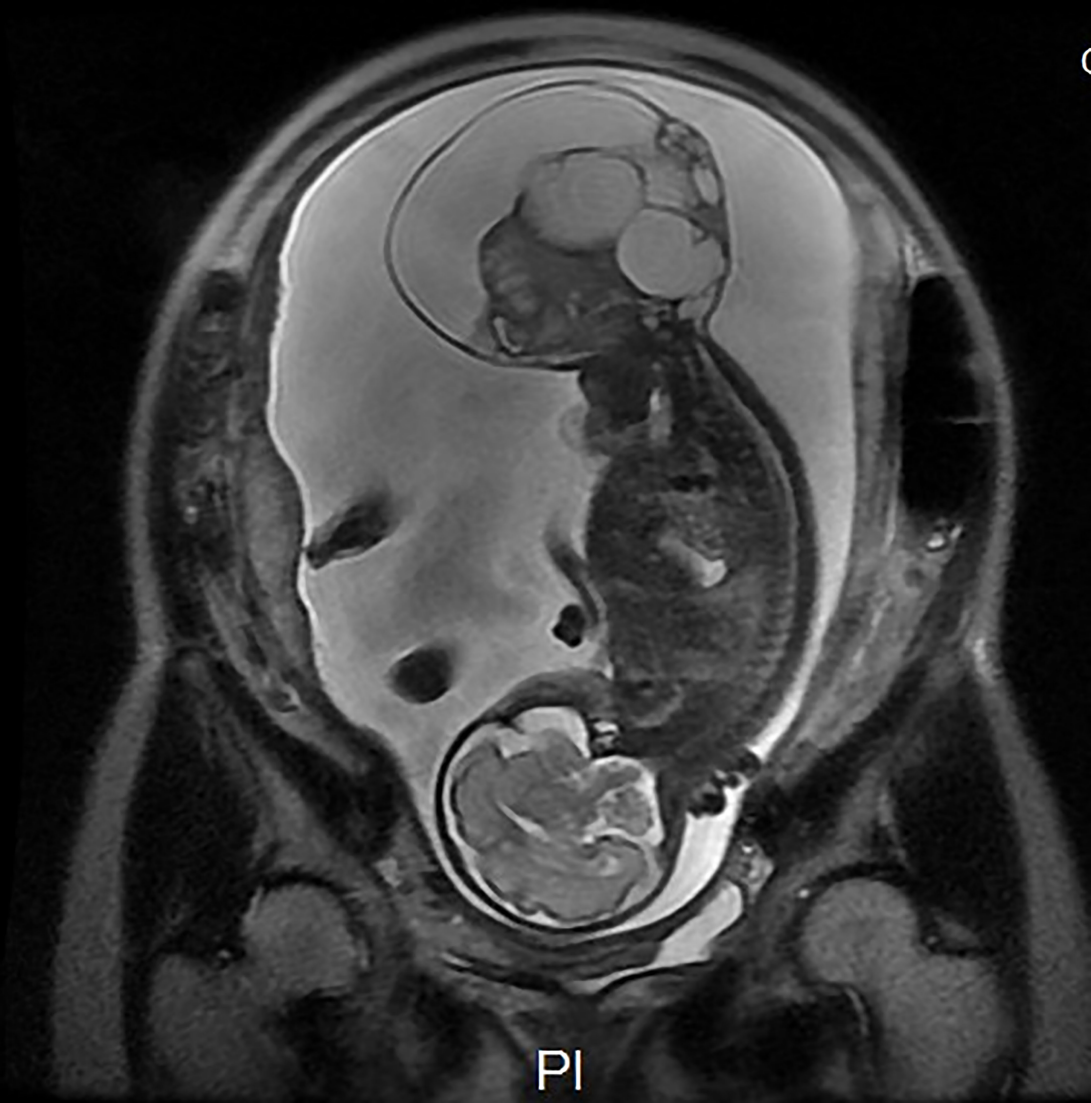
Magnetic resonance imaging was performed at 27 weeks. There was no evidence of possible invasion to the foetal pelvis or abdomen. The intact spine, foetal kidneys, and bladder were normal. A diagnosis of type I in the Altman classification was confirmed. Ax DWI b = 600.
